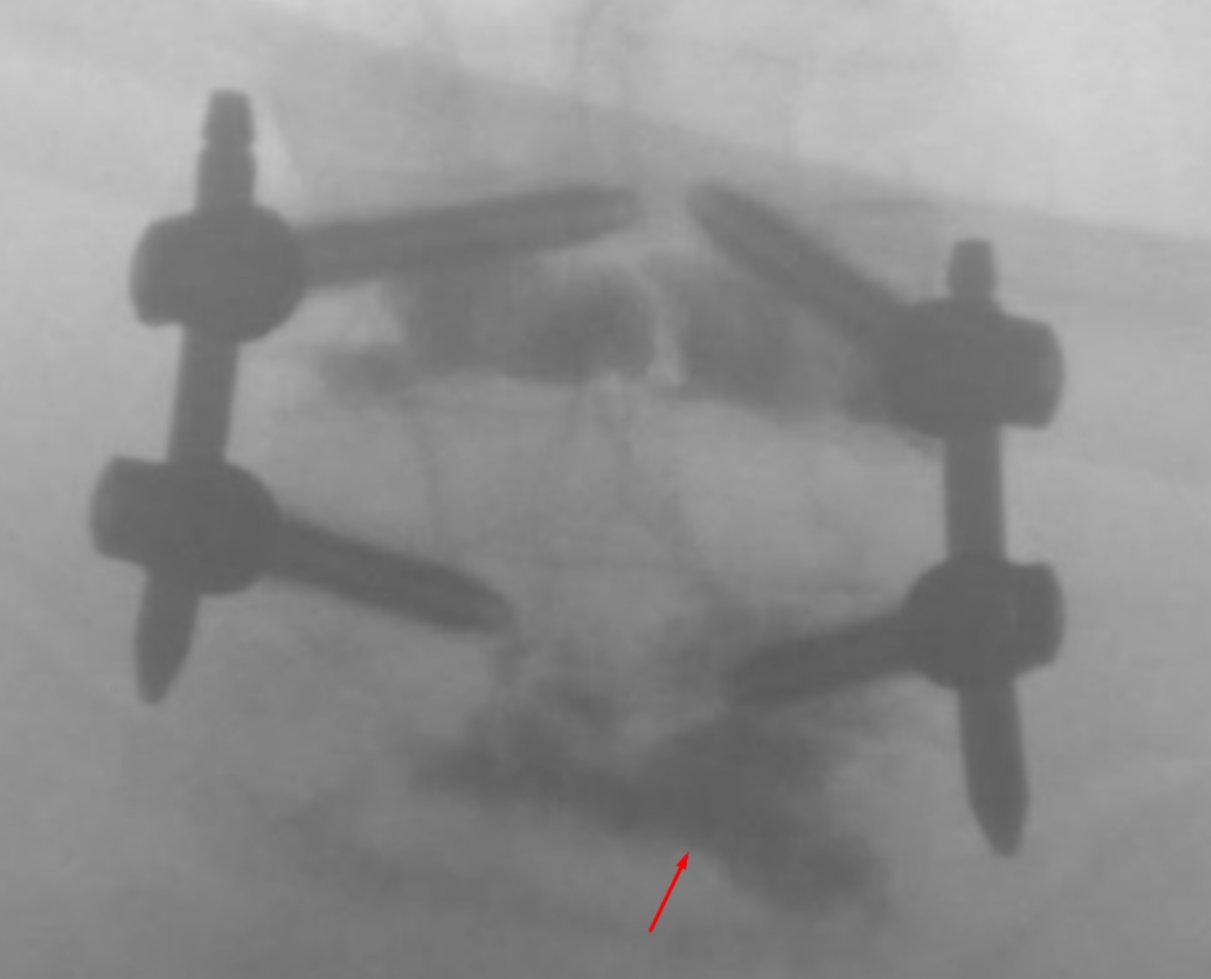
AP View - Fixation Final anterior-posterior (AP) fluoroscopic X-rays demonstrating final fixation of the bone cement. Arrow indicates the bone cement.
